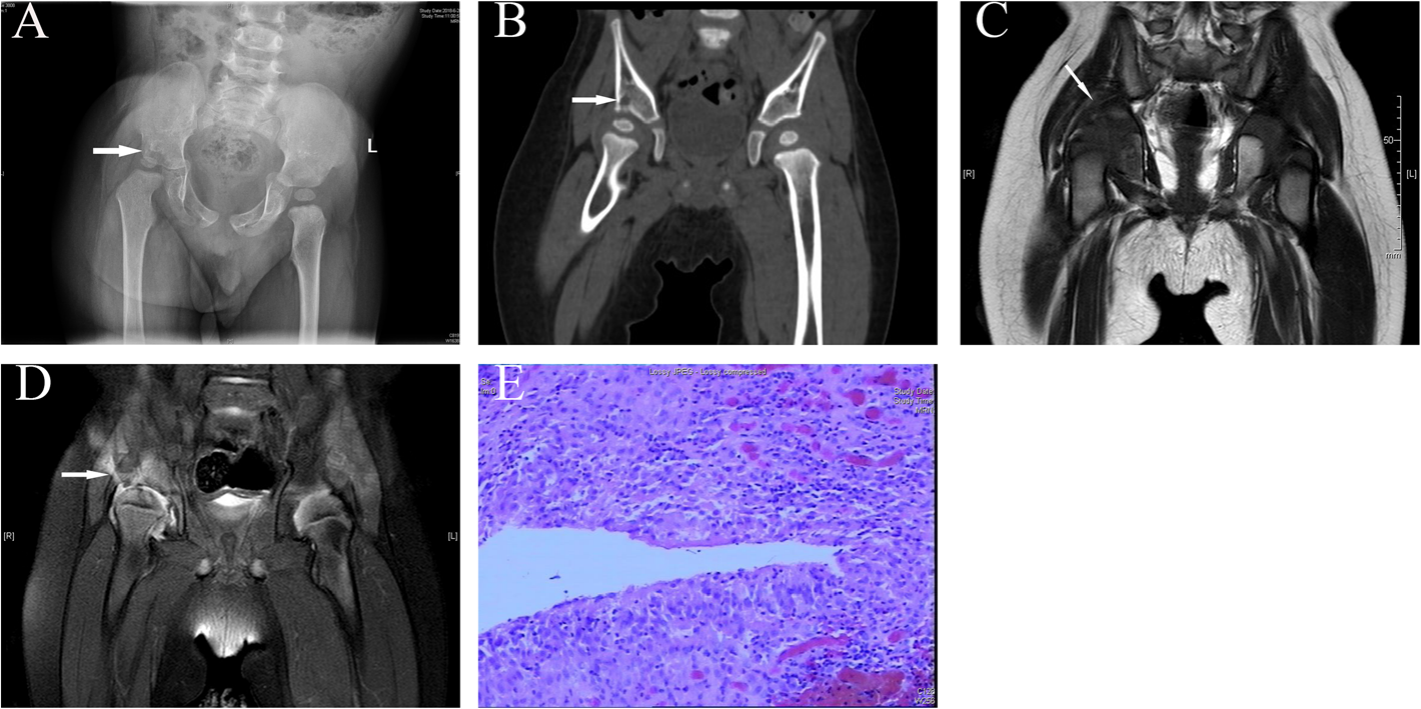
a-e A 2.9-year-old male patient (case J). The X-ray and CT results show right acetabular bone destruction, with a low-density shadow and hip subluxation (a, b). MRI shows patchy, slightly long T1 and slightly long T2 signals in the right ischial and iliac bone and long T2 signals in the right hip joint space (c, d). The pathological results suggest chronic inflammatory cell infiltration and granulomatous inflammation with a multinucleated giant cell response (e) (white arrows indicate the site of the lesion)
